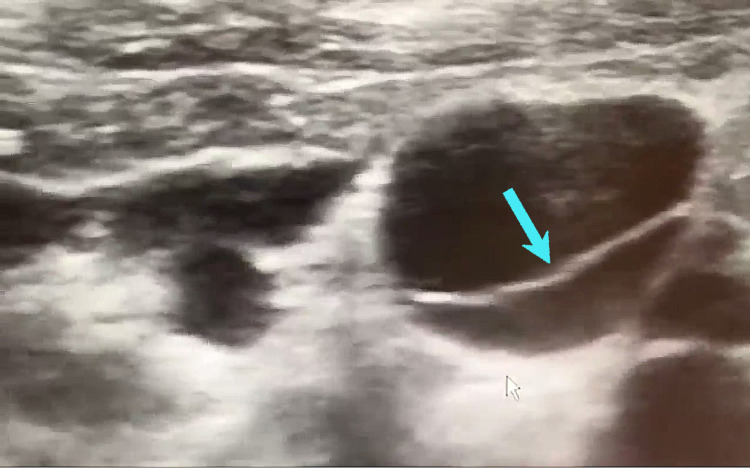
Axial greyscale Right internal jugular vein ultrasound image. Axial greyscale right internal jugular vein ultrasound image showing a right internal jugular vein complete valve as a thin echogenic linear structure in the central lumen at the level of the thoracic inlet (arrow). The valve is of the bicuspid type, in which its motion throughout the cardiac cycle can be appreciated, as seen in Video 1.
